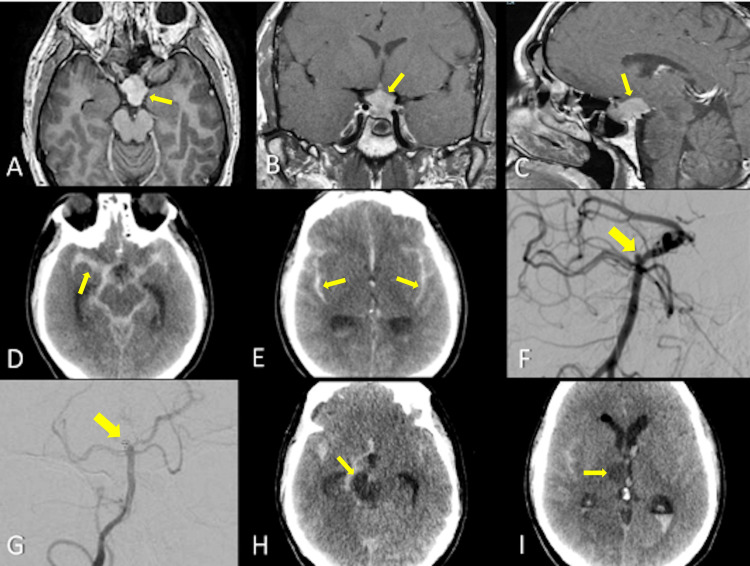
Case 1 representative MRI, CT, and DSA images. (A-C) MRI shows a sellar tumor compatible with pituitary adenoma bulging into the mesencephalic cistern (arrows). (D,E) PO CT displaying a massive subarachnoid hemorrhage extending into the peri-mesencephalic cisterns (arrows). (F) A small basilar top aneurysm was discovered during the DSA (arrow). (G) The aneurysm was coiled for dome protection (arrow). (H,I) CT at PO day 12. Low-density areas appeared at mesencephalic and thalamic areas (arrows). MRI, magnetic resonance imaging; CT, computed tomography; DSA, digital subtraction angiography; PO, postoperative.
